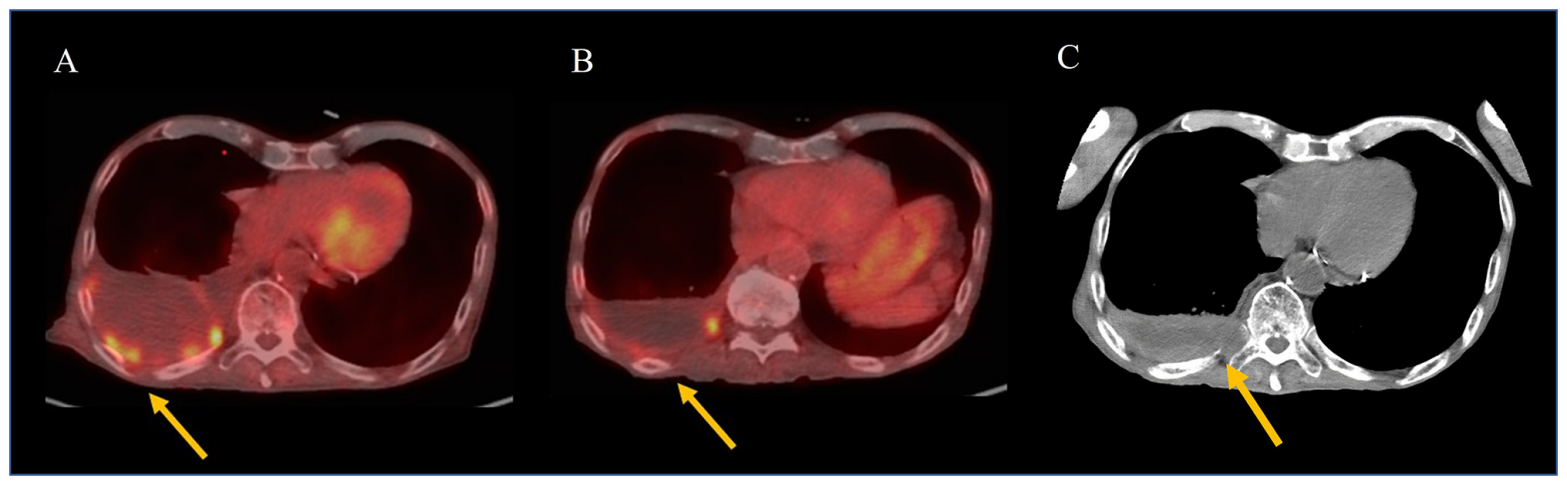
(A and B) Positron emission tomography/CT and (C) CT images of Case 3. (A) Case 3 before nivolumab treatment. The yellow arrow indicates FDG uptake in the pleural thickening. (B) After two cycles, the right pleural thickening and effusion (yellow arrow) improved, and FDG uptake decreased. (C) At 6 months after the end of treatment, the pleural thickening worsened again (yellow arrow), and invasion of the pleura and ribs was observed. FDG, ^18^F-fluorodeoxyglucose.
